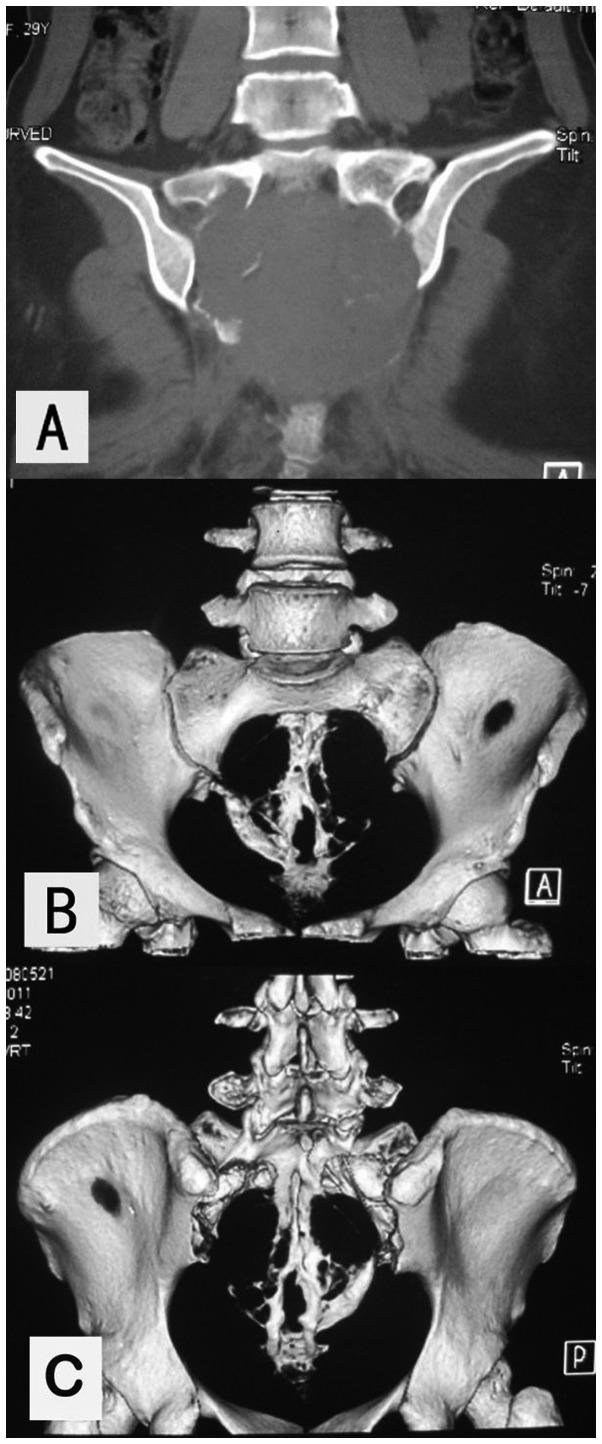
(A) Computed tomography (CT) scan and (B and C) three-dimensional reconstruction showing osteolytic destruction of the sacrum and a large soft-tissue mass involving the underlying left ilium. (B) Three-dimensional scan viewed from the front. (C) Three-dimensional scan viewed from behind. The sacral canal was enlarged and the left medial ilium (near sacroiliac joints) was destroyed.
